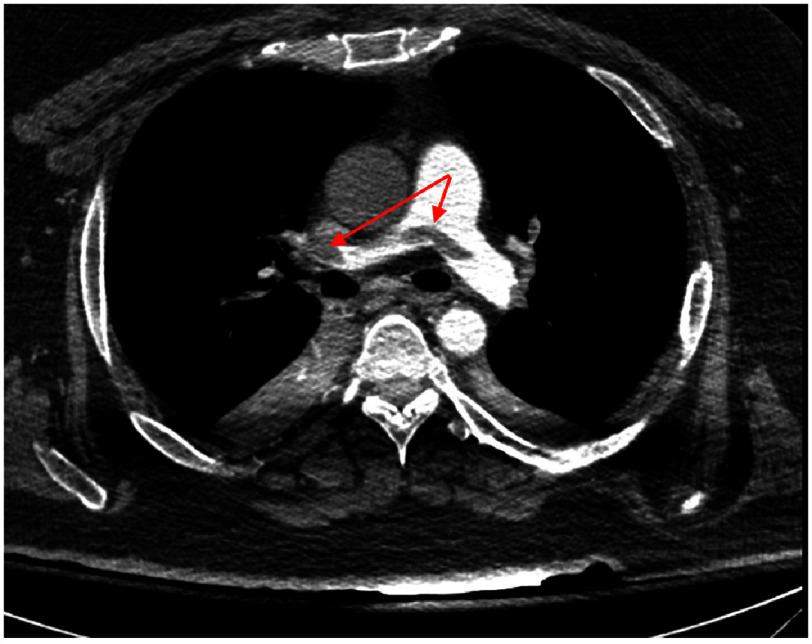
CTA of the chest revealing saddle thromboembolism in the main pulmonary artery and right upper lobar pulmonary artery (arrows).
